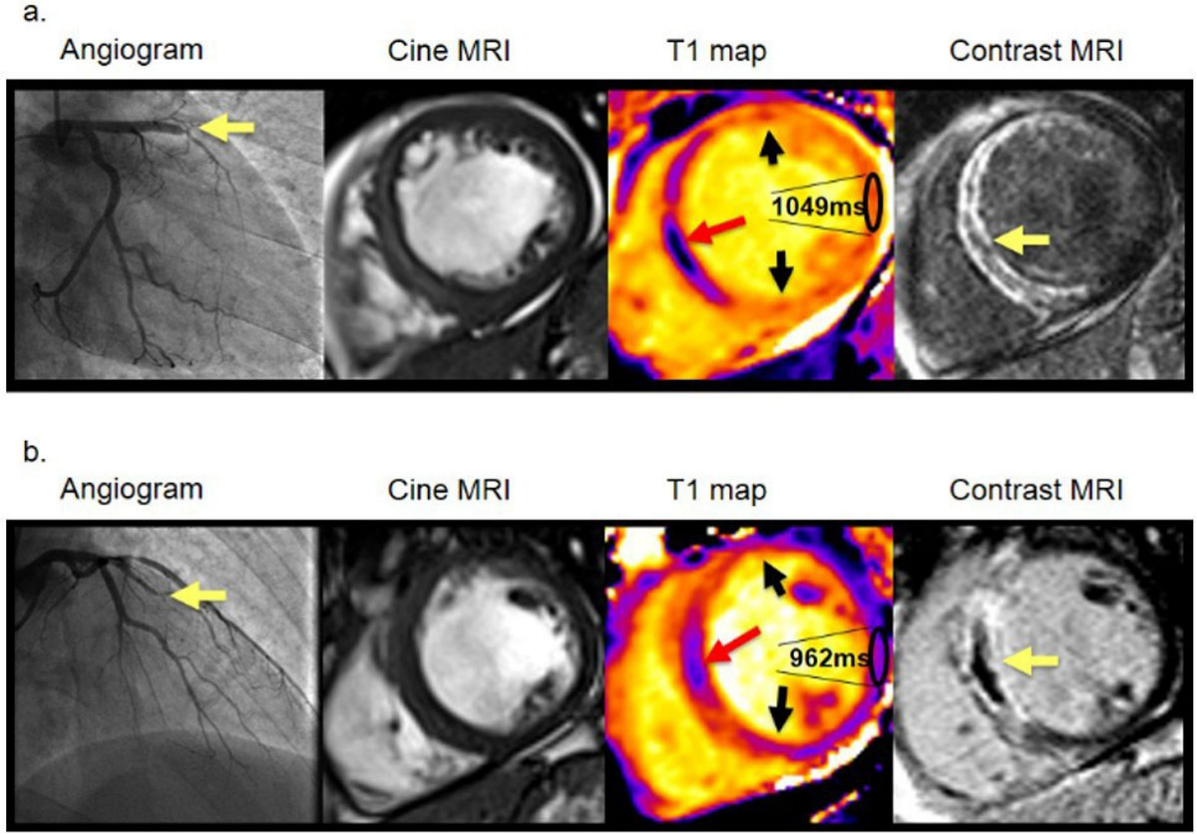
Two patients with acute anterior STEMI treated by primary PCI and with the same standard anti-thrombotic therapies. Each patient had TIMI grade 3 flow at the end of the procedure. (*a*) *Patient with high remote zone native T1.* Six month follow-up MRI revealed final infarct size was 39.2% of left ventricular mass and significant adverse remodeling occurred with left ventricular end-diastolic volume of 145.7 ml/m2. This patient was subsequently hospitalised for new onset heart failure and had an defibrillator device implanted. (*b*) *Patient with average remote zone native T1 value.* The infarct size at 6 months revealed by contrast-enhanced MRI was 31.0% of left ventricular mass and left ventricular end-diastolic volume of 84.3 ml/m2. This patient had an uncomplicated clinical course.
